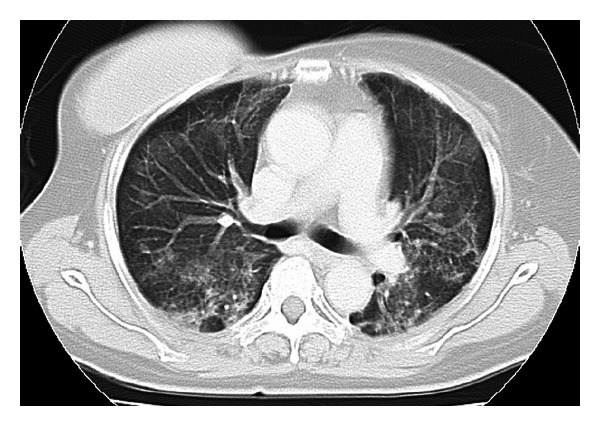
Computed tomography at the time when our patient complained of severe dyspnea. CT of the chest revealing widespread patchy ground glass changes in bilateral lobes.
